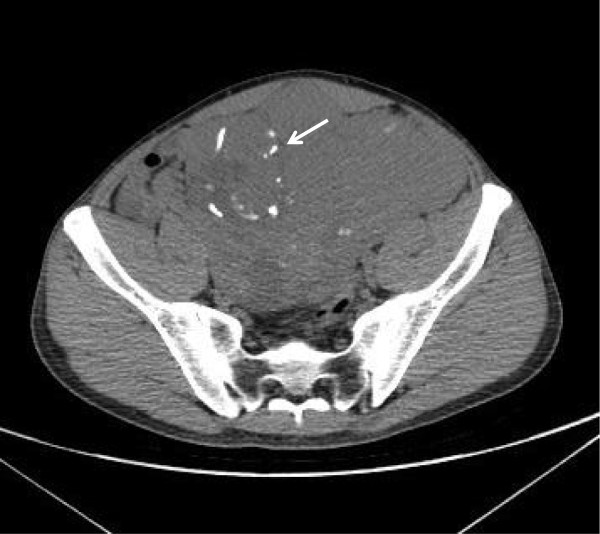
Pelvic enhanced computed tomography that shows a 10×9×7 cm mass with heterogeneous density and calcifications (Arrow).
